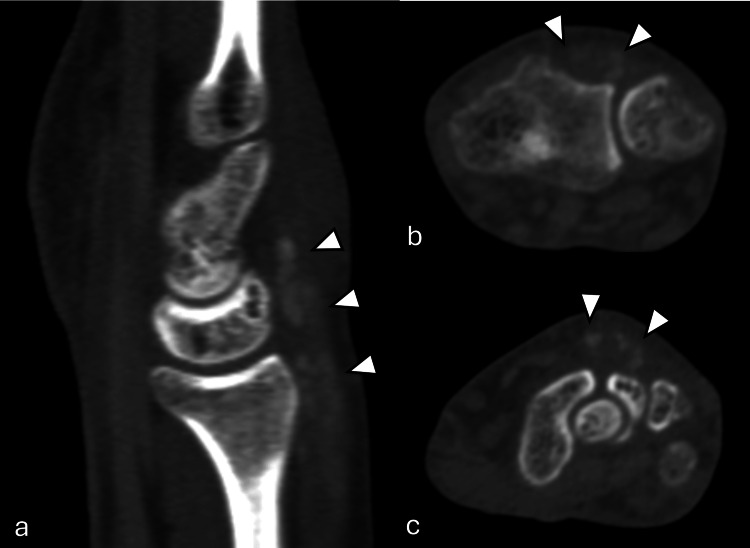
CT right wrist in bone window CT right wrist in bone window with sagittal (a) and axial (b, c) planes. Soft tissue swelling with high-density foci (arrowheads) over the dorsal aspect of the wrist, involving the extensor tendons, corresponds to intratendinous gouty tophi CT: computed tomography
